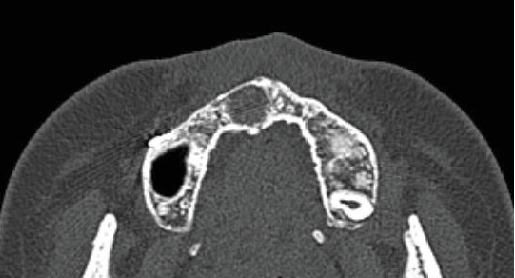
CT obtained 1 year after surgery shows no signs of recurrence.
